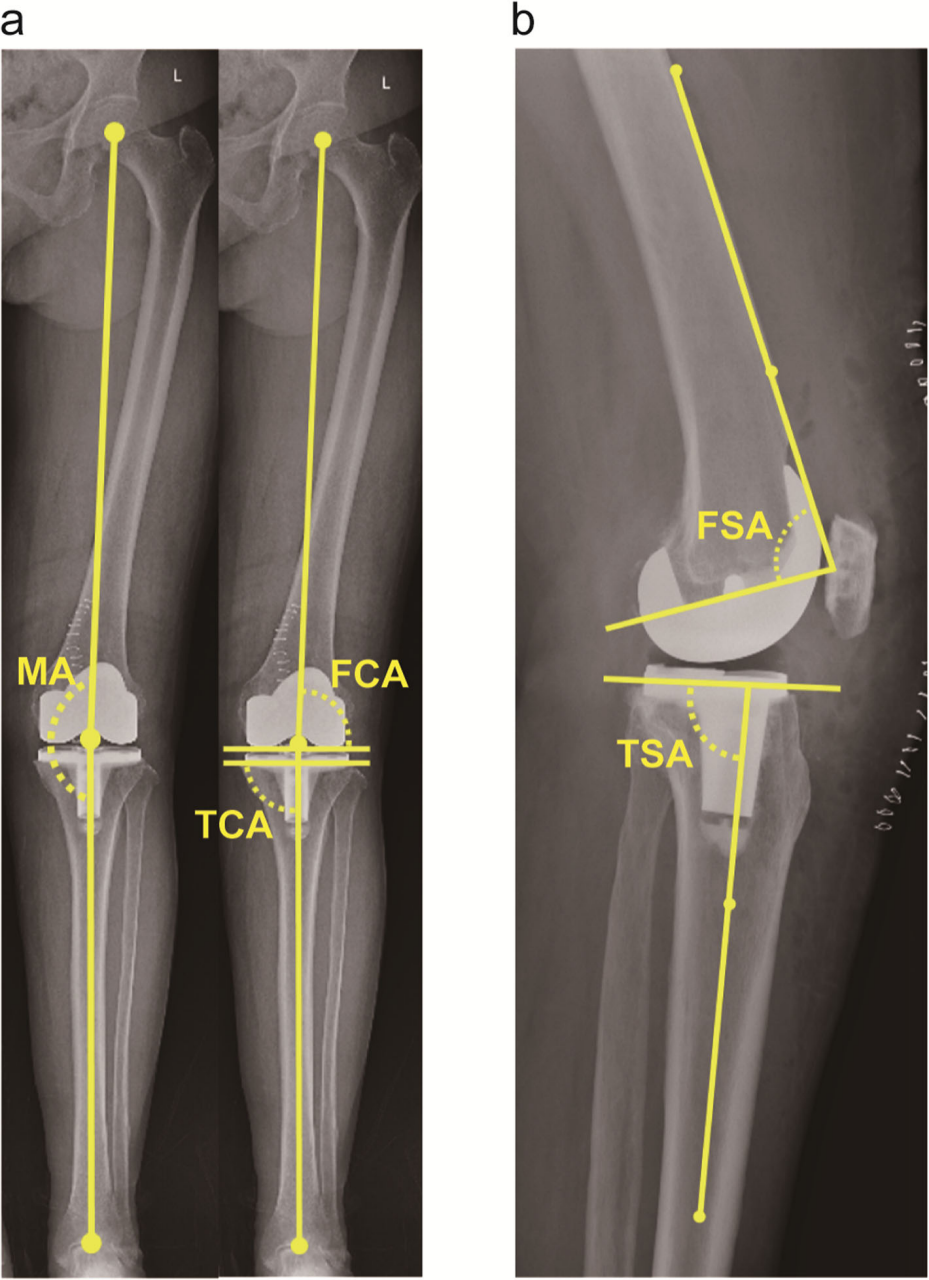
a Long-leg standing AP film for radiographic measurements in the coronal plane, including MA, FCA, and TCA. b Lateral radiograph for measuring component alignments in the sagittal plane, including FSA and TSA. Abbreviations, MA, mechanical axis; FCA, femoral coronal angle; TCA, tibial coronal angle; FSA, femoral sagittal angle; TSA, tibial sagittal angle
